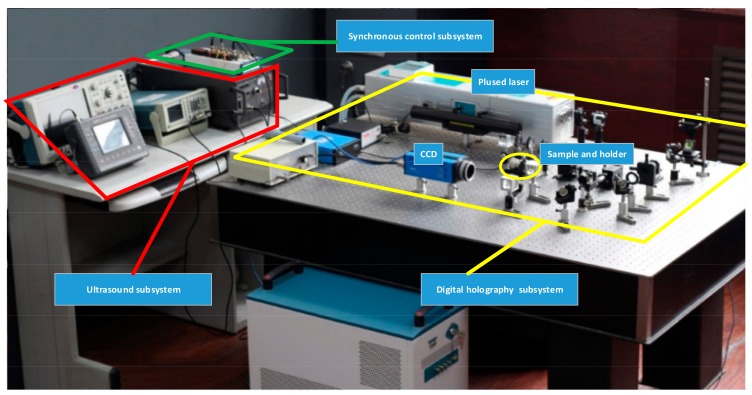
The proposed 3D Ultrasonic Wavefields measurement system.
